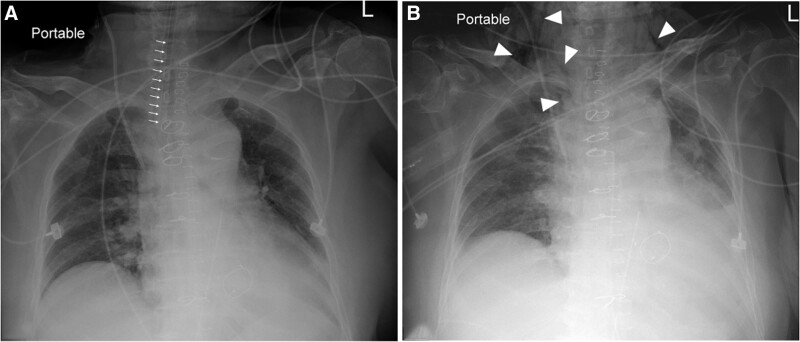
Portable chest radiographs taken on anterioposterior view. (A) Day 1 after surgery and (B) Six hours after application of high-flow nasal oxygenation following removal of endotracheal tube. Arrows indicate the position of endotracheal tube on chest radiograph of postoperative day 1. Arrowheads indicate subcutaneous emphysema in the bilateral neck and supraclavicular regions. No signs of pneumothorax or pneumomediastinum were found on these chest radiographs.
